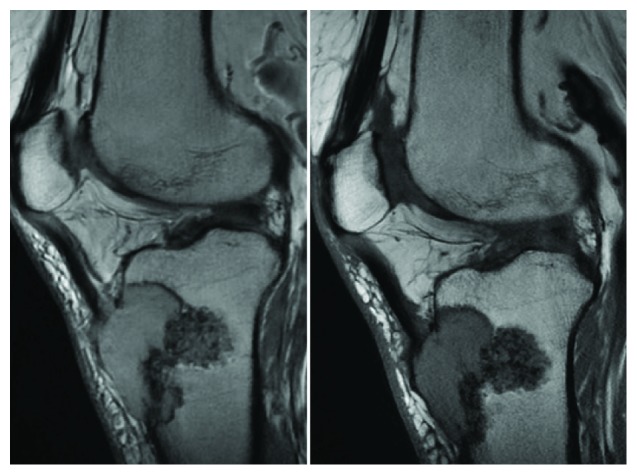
MRI of the right knee: sagittal T1-WI and T2-WI sequences of the knee show a 4.5 cm lytic expansile mass at the anterior proximal aspect of the tibia involving the patellar tendon insertion. A sclerotic area is seen along the posterior border.
